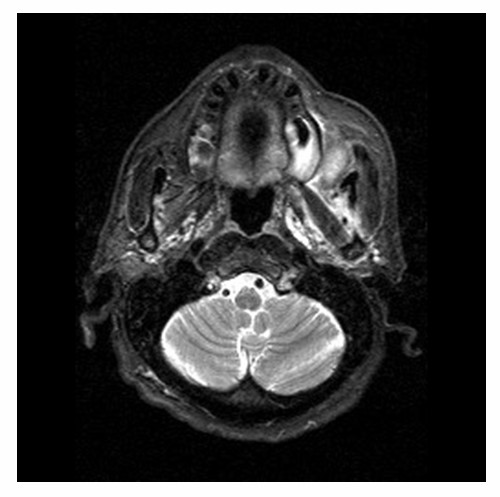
Axial MRI imaging of the tumor. The margin of the lesion was clear, no invasion was observed.
